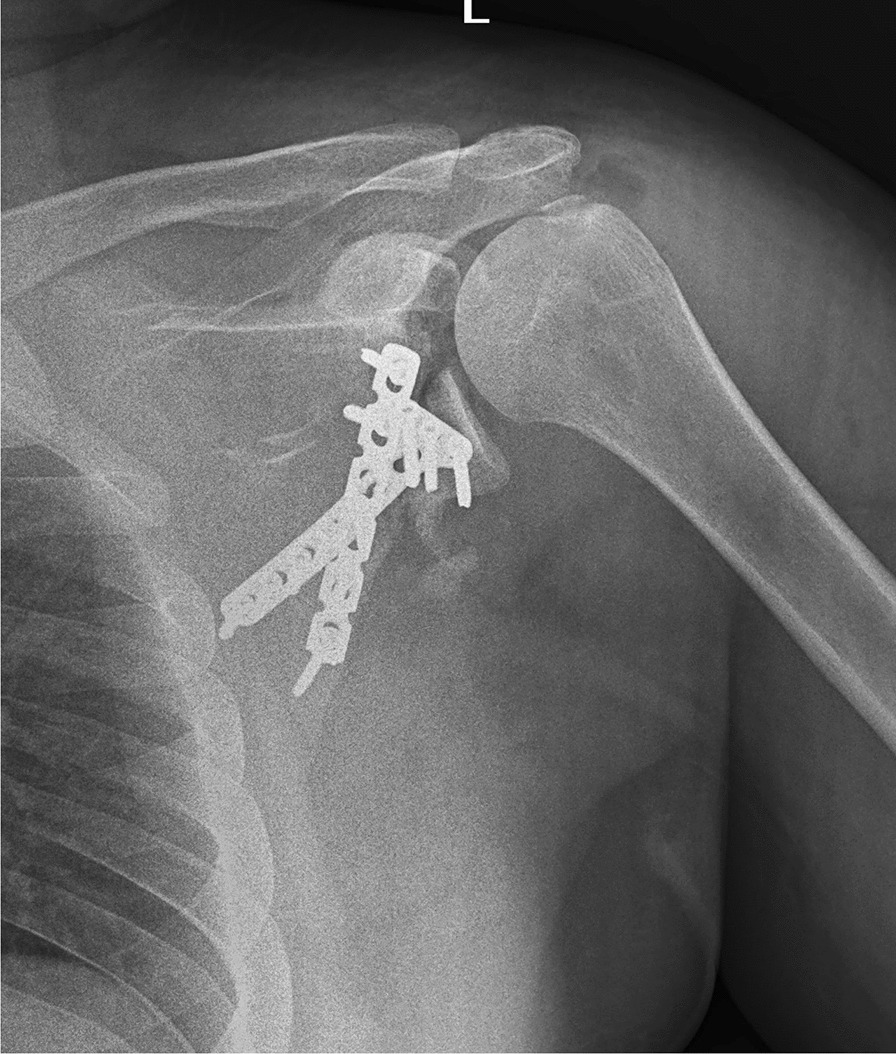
Postoperative shoulder X-ray shows the utilization of two reconstruction and T 3.5 mm plates to fixation of the scapula anatomical neck fracture
